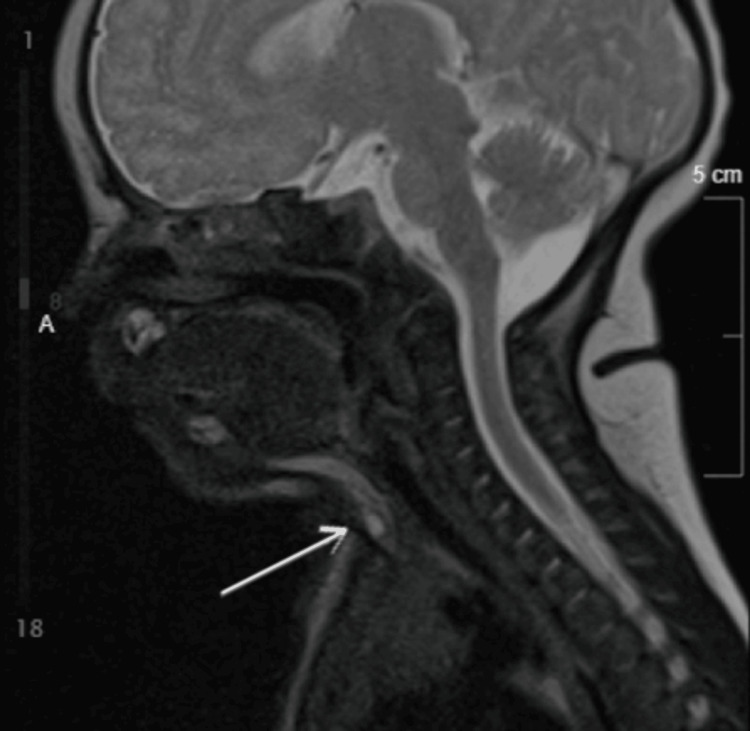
Sagittal MRI of the neck (additional sequence) demonstrating a superficial infrahyoid midline lesion (arrow) without deep extension, consistent with congenital midline cervical cleft. MRI: magnetic resonance imaging
